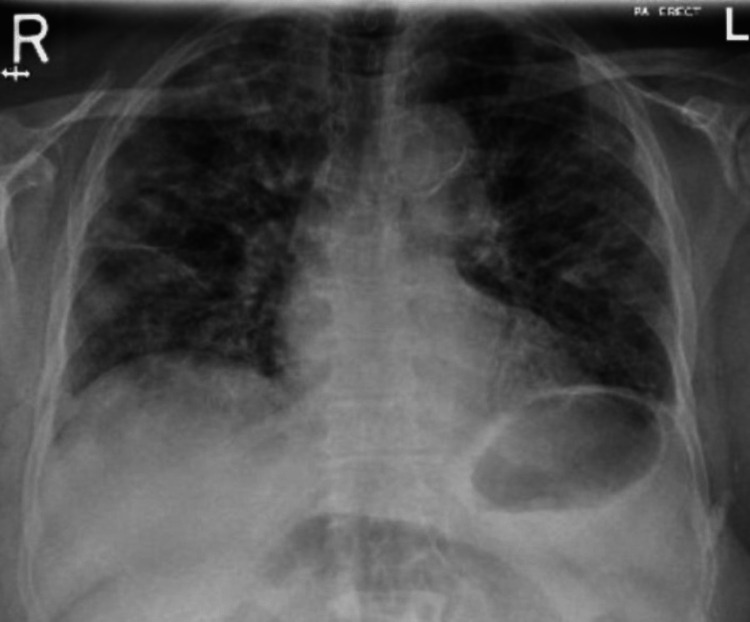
Chest X-ray showing bilateral reticular infiltrates with patchy ground glass opacities
